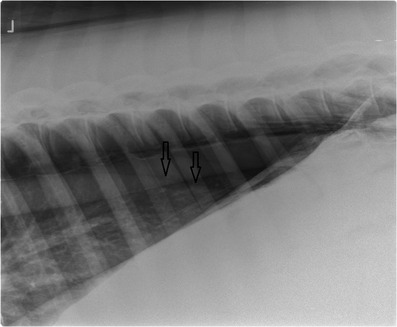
Left latero‐lateral radiograph of the caudodorsal thorax; cranial is to the left, image from day 7. Arrows outline the caudodorsal margins of the left and right collapsed lung lobes consistent with bilateral pneumothorax. The triangular radiolucent area in the caudodorsal pleural cavity is smaller than in the radiograph shown in Figure 2
